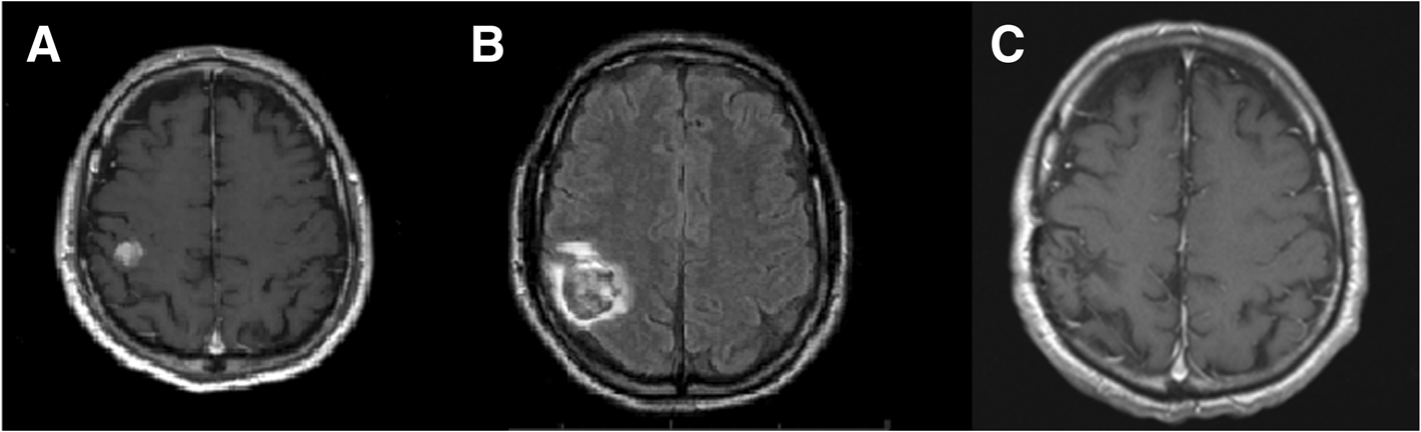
Coronal contrast-enhanced MRI brain images showing (a) initial 1.3 cm right parietal lobe metastasis without mass effect or cerebral edema; (b) enlarging 4 cm hemorrhagic right parietal mass with vasogenic edema 6 weeks post-gamma knife; (c) no evidence of brain metastases 12 years after resection
